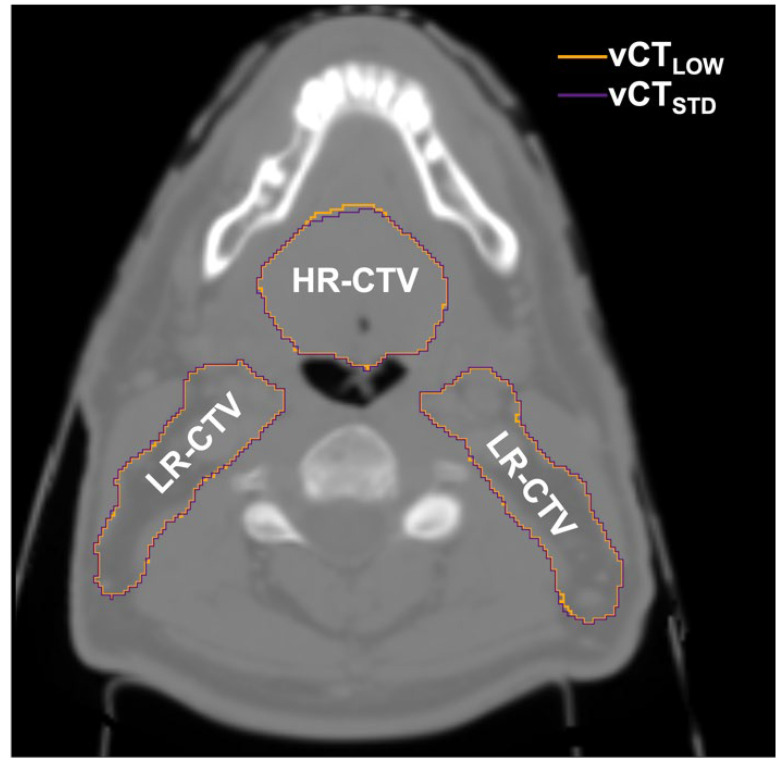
Difference between contours propagated to the standard-protocol CT (vCT_STD_) and the lowest-dose CT (vCT_LOW_) for the high-risk CTV (HR-CTV) and low-risk CTV (LR-CTV).
